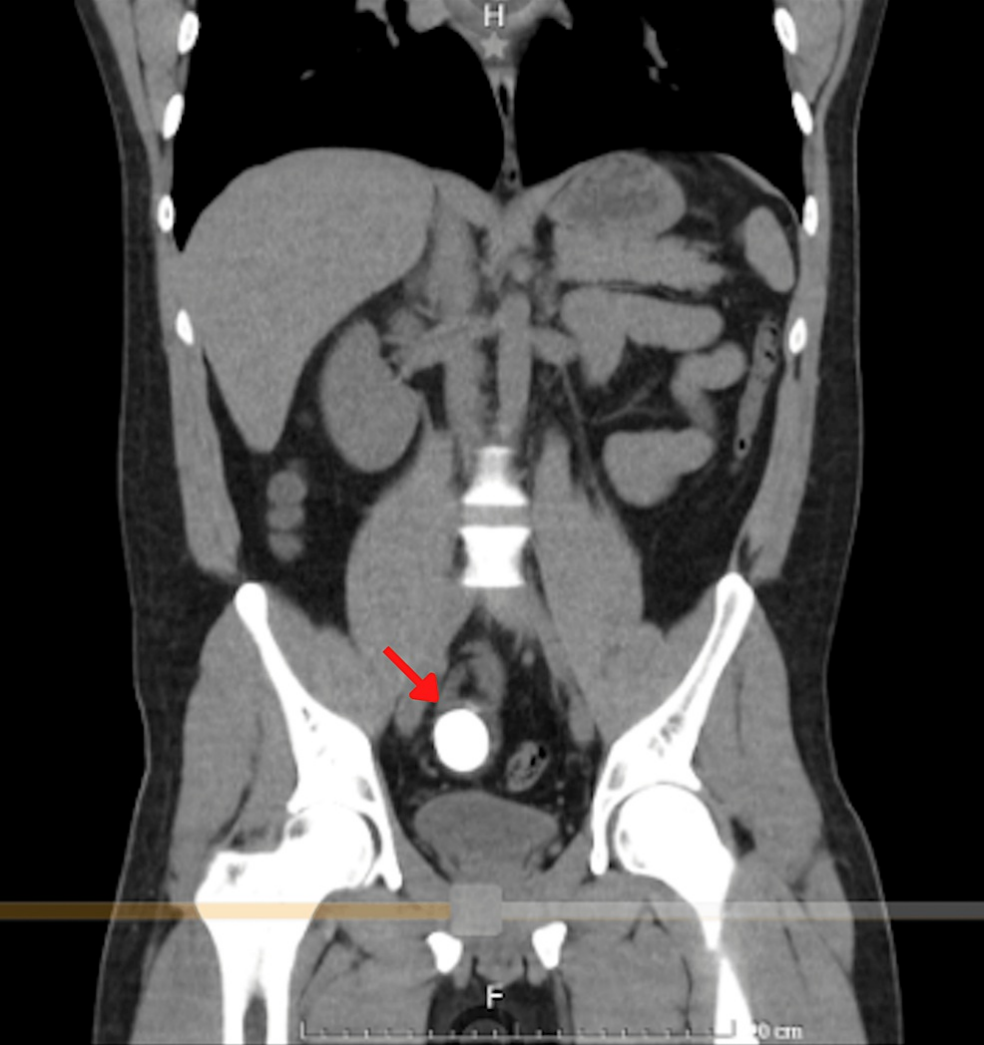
Coronal view of abdominal computed tomography revealing the gallstone (the red arrow) in the distal ileum.
